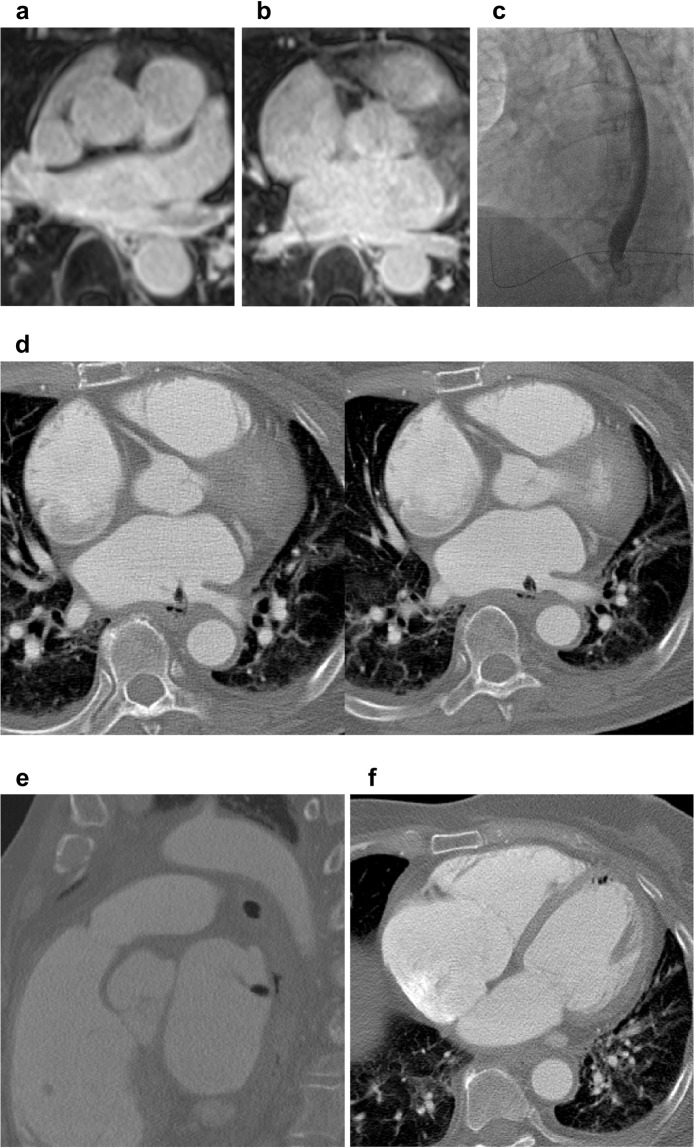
Atrio-esophageal fistula with massive air leakage. (a–c) Pre-procedure imaging studies revealed that esophagus was immediately adjacent to left inferior pulmonary vein. (d,e) CT scan revealed a definite atrio-esophageal fistula near left inferior pulmonary vein and significant amount of air leakage. (f) Free air was also observed in the left ventricle. CT: computed tomography.
